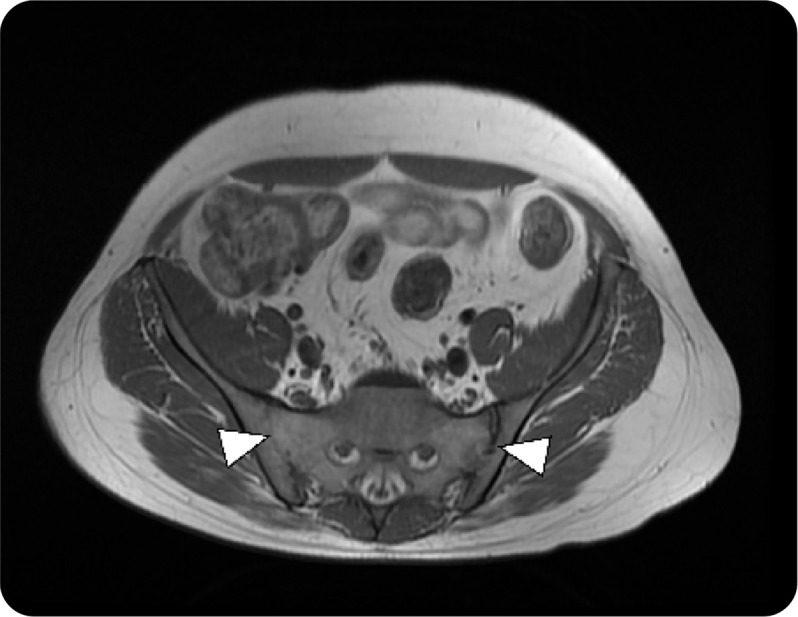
(above). T1W MRI of P3 demonstrating bilateral ankylosis (White arrows).
